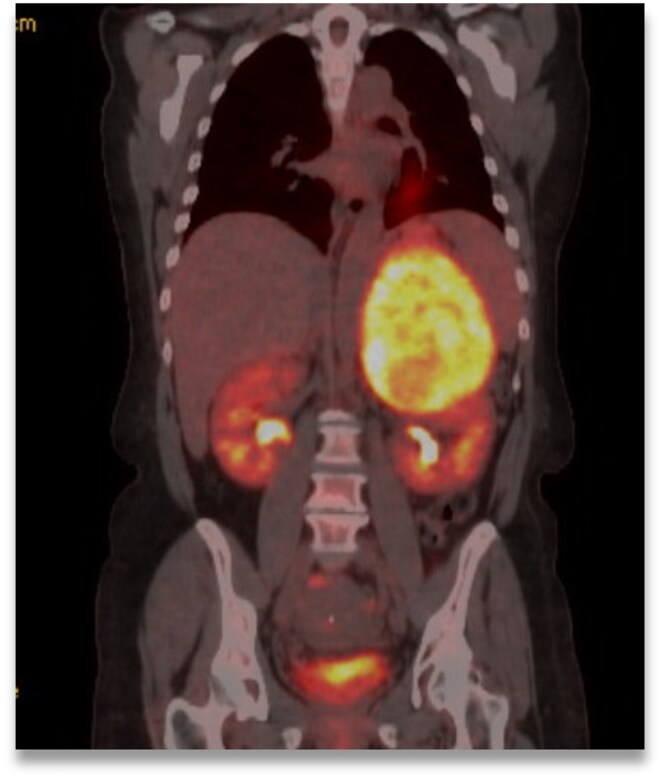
FDG-PET, coronal view, showing FDG-avid left-sided adrenal lesion.
